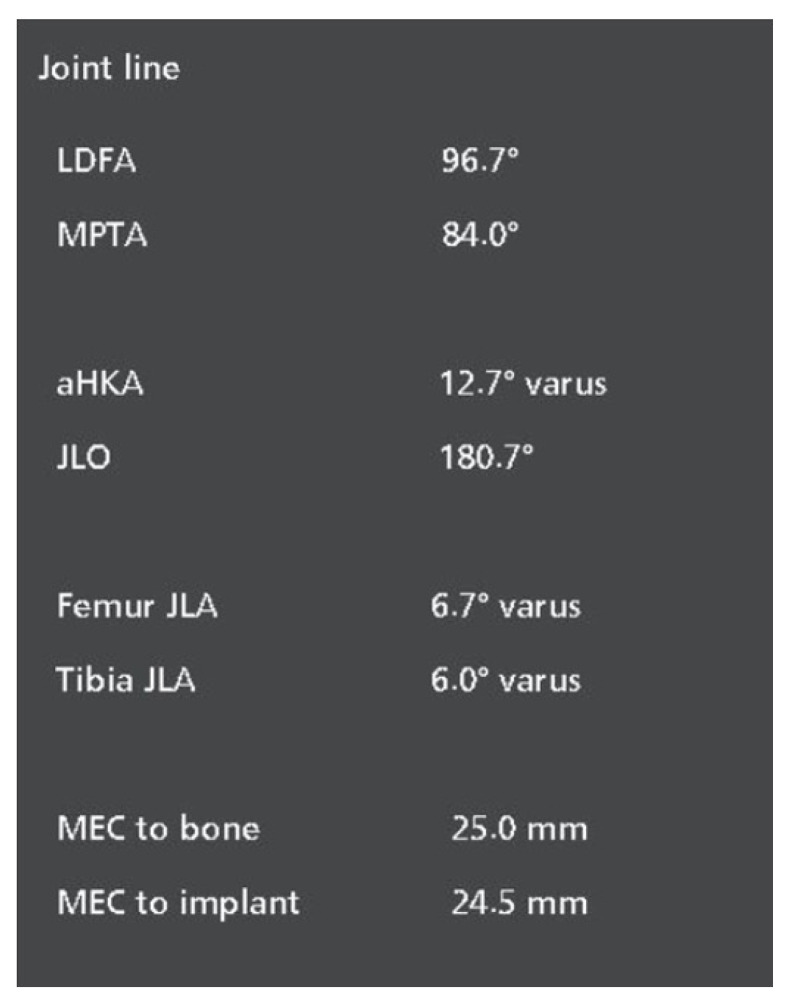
Software-generated measurements available to the surgeon upon the successful upload, segmentation and verification of the pre-operative CT scan.
